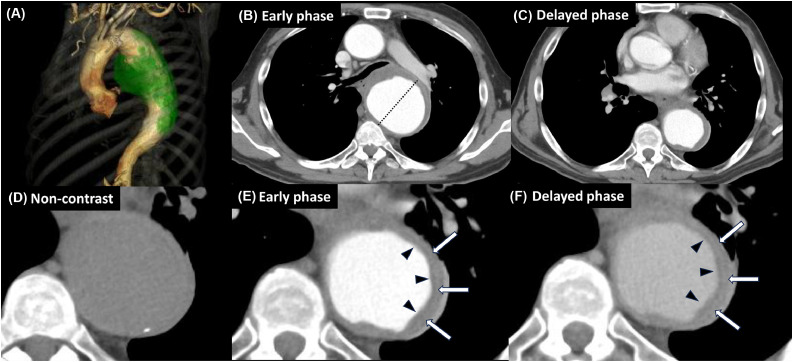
(A) Volume-rendered image. (B, C) Early-phase images. (D–F) Magnified views of (C), including non-contrast (D), early phase (E), and delayed phase (F). Contrast-enhanced CT demonstrates a descending thoracic aortic aneurysm with circumferential wall thickening. The intimal layer shows poor enhancement (arrow head), whereas the outer medial and adventitial layers demonstrate ring-like enhancement (arrow) (double-ring sign), suggestive of inflammatory aortitis. The aneurysm measured 70 mm in maximum diameter (dotted line).
